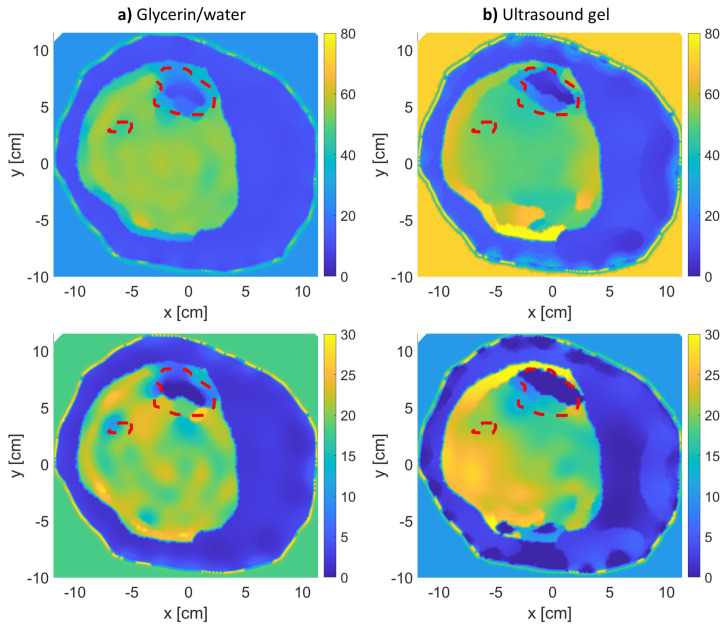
The real (top row) and imaginary (bottom row) relative complex permittivity results for leg model 3 with the incorporation of prior information about the fat layer for two different matching media: (a) glycerin/water solution and (b) ultrasound gel.
